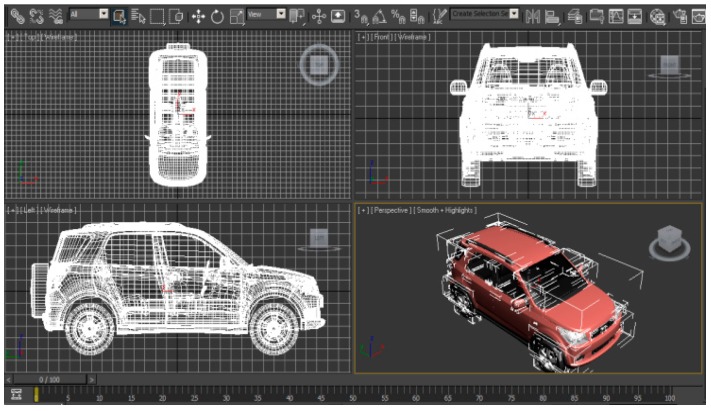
Model of a Small SUV for RCS calculation.
